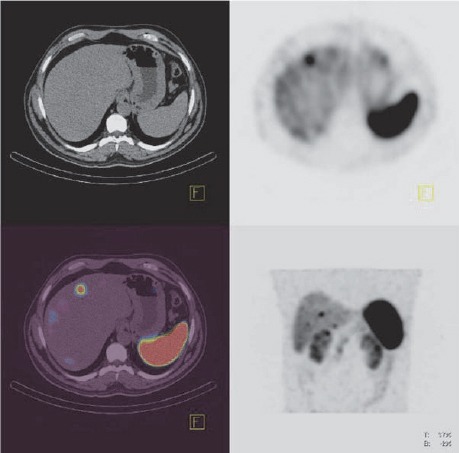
Transaxial SPECT, CT and fused SPECT/CT as well as coronal image shows uptake in the duodenum and liver lesions.
